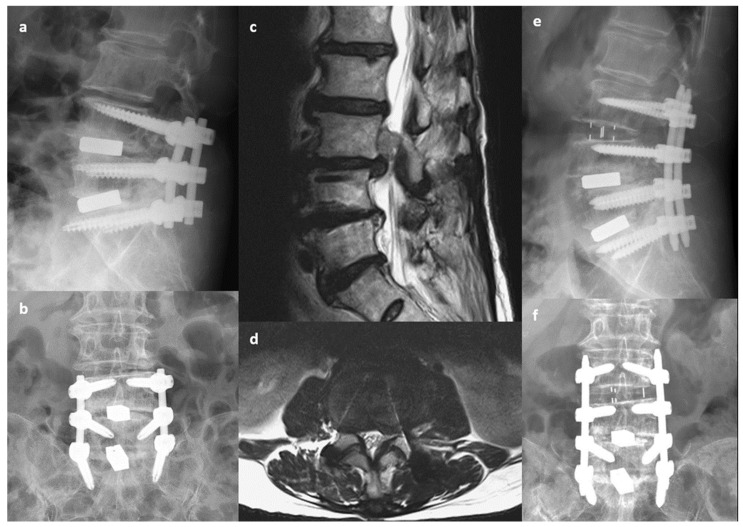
A 78-year-old woman with low back pain and right thigh radiation. Primary transforaminal lumbar interbody fusion was performed 3 years previously to treat spinal stenosis and disc herniation at L4-5-S1. (a,b) Preoperative X-ray indicating decreased disc height at L3-4. (c,d) Preoperative MRI indicating uncontained disc extrusion with upper migration at L3-4. (e,f) Postoperative X-ray indicating oblique lumbar interbody fusion at L3-4 with extension of posterior instrumentation to L3. Left-side laminotomy of L3 was performed to remove the migrated disc.
